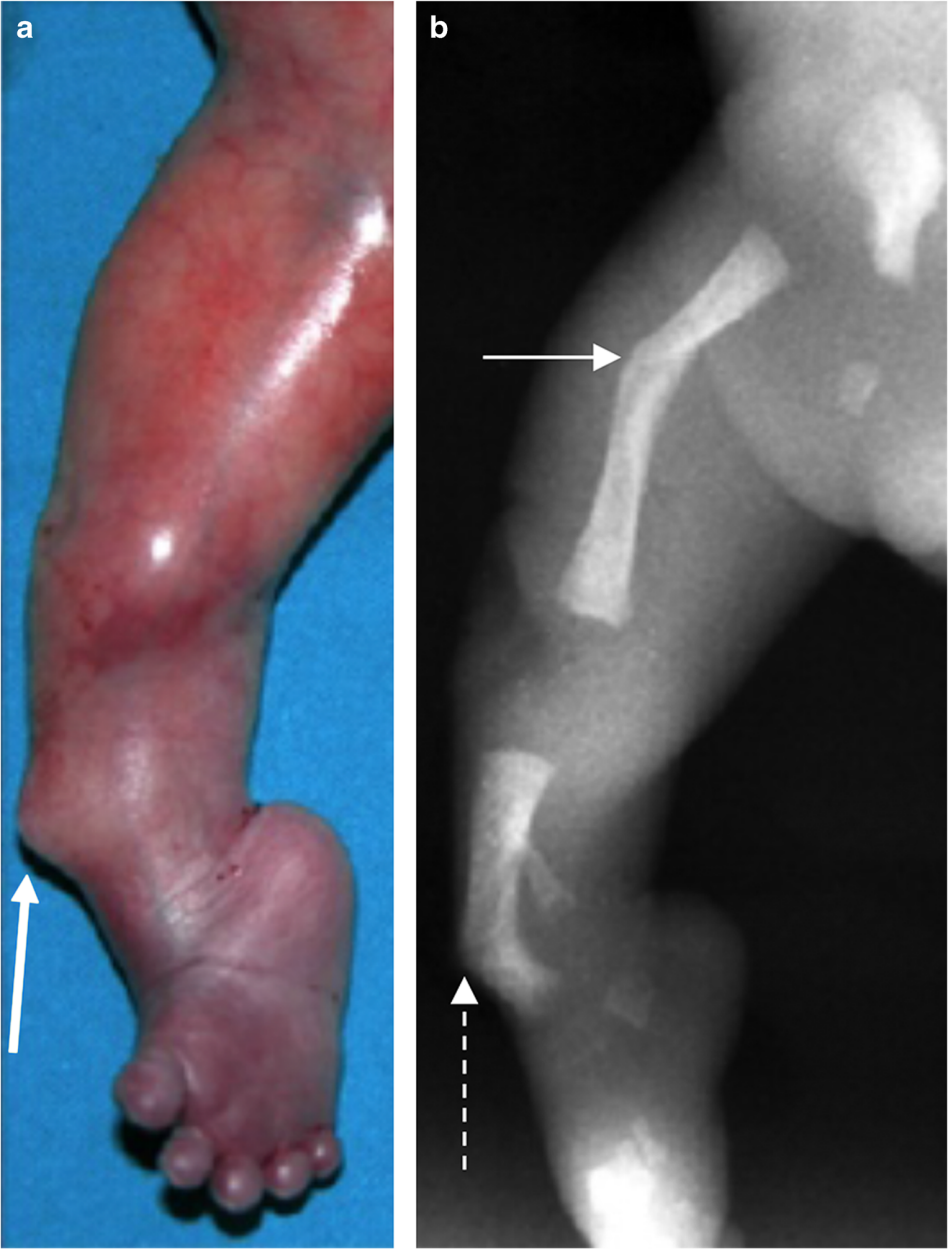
Clinical and radiographic features of lethal campomelic dysplasia in a male neonate. a,b Photograph (a) and radiograph (b) show angulation at the junction of the proximal third and distal two-thirds of the femur (*arrow*) and the junction of the proximal two-thirds and the distal third of the tibia (*dashed arrow*). Note the clinical spur (*yellow arrow*), which may also be seen in hypophosphatasia. [Images reproduced with permission from Radcliffe Publishing (38), page 246, case 1, image 1c]
